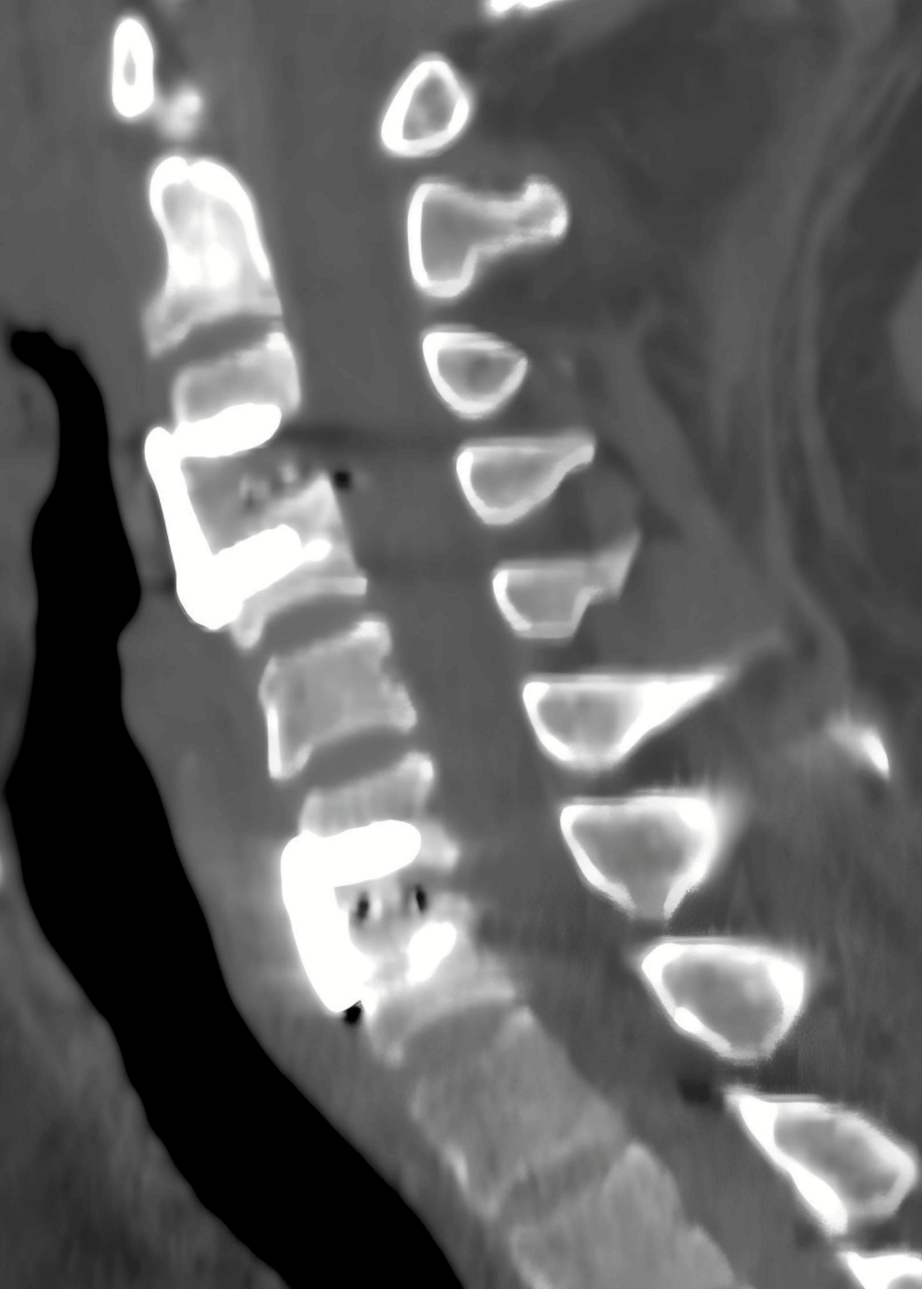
CT scan three months after the surgery Six months after the operation, the CT scan showed no hematoma, the internal fixation was in good position, and fusion had occurred.
